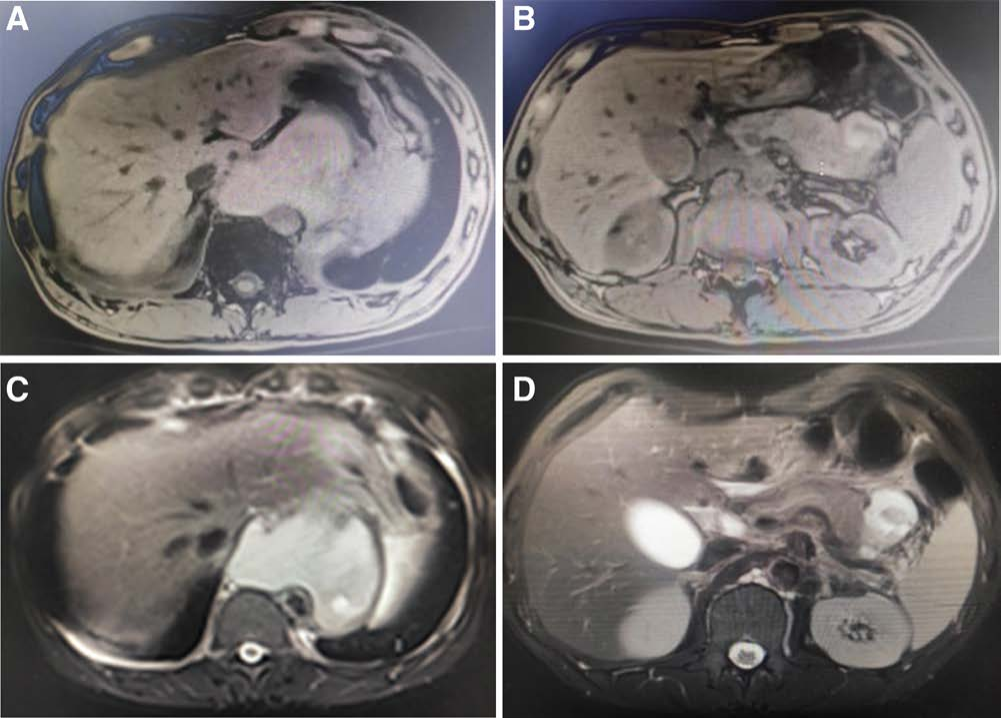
Abdominal MRI (following thoracentesis and drainage): encapsulated fluid in the pancreatic tail-posterior mediastinal area, which is indicative of a pancreatic pseudocyst herniating into the mediastinum. (A, B) T1-weighted image; (C, D) T2-weighted image. MRI = magnetic resonance imaging.
